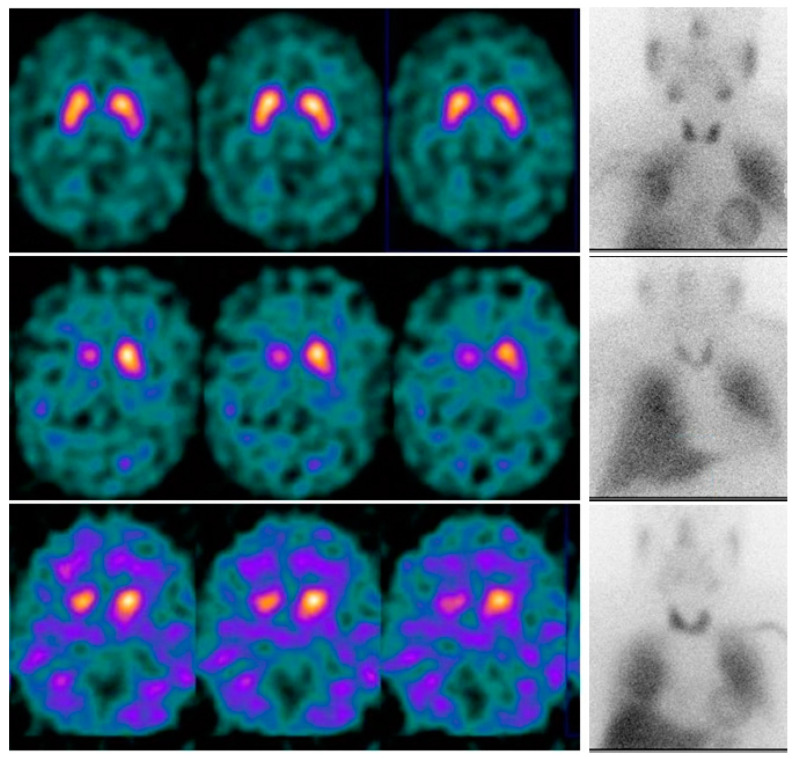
Combination of [^123^I]Ioflupane SPECT and [^123^I]MIBG scintigraphy in the study of parkinsonism. Top row: healthy patient: preserved uptake in both striatal nuclei and integrity of myocardial sympathetic function. Middle row: patient with PD: bilateral asymmetric striatal hypocaptation and absence of myocardial sympathetic function. Bottom row: patient with MSA-P: bilateral striatal hypocaptation (more symmetrical) with marked presence of cortical nonspecific activity and intact or slightly decreased myocardial sympathetic function.
